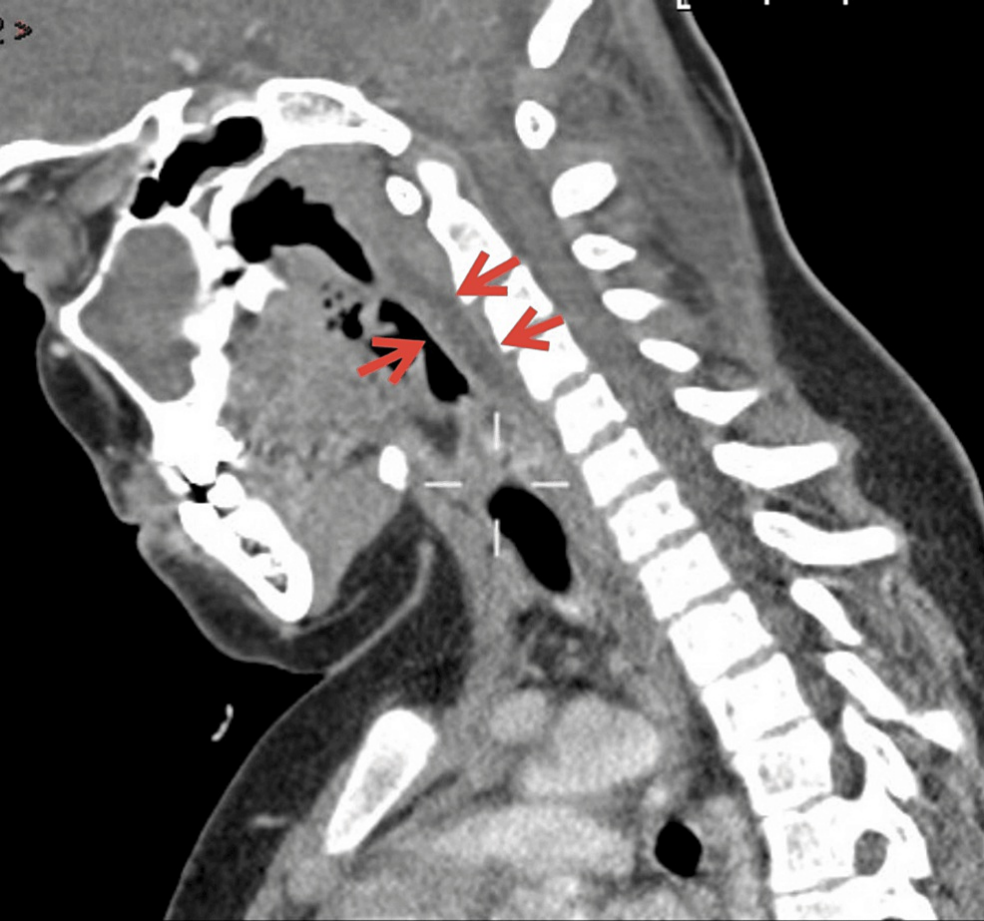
Contrast CT of the neck sagittal view. The red arrows indicate the presence of prevertebral or retropharyngeal collection.
